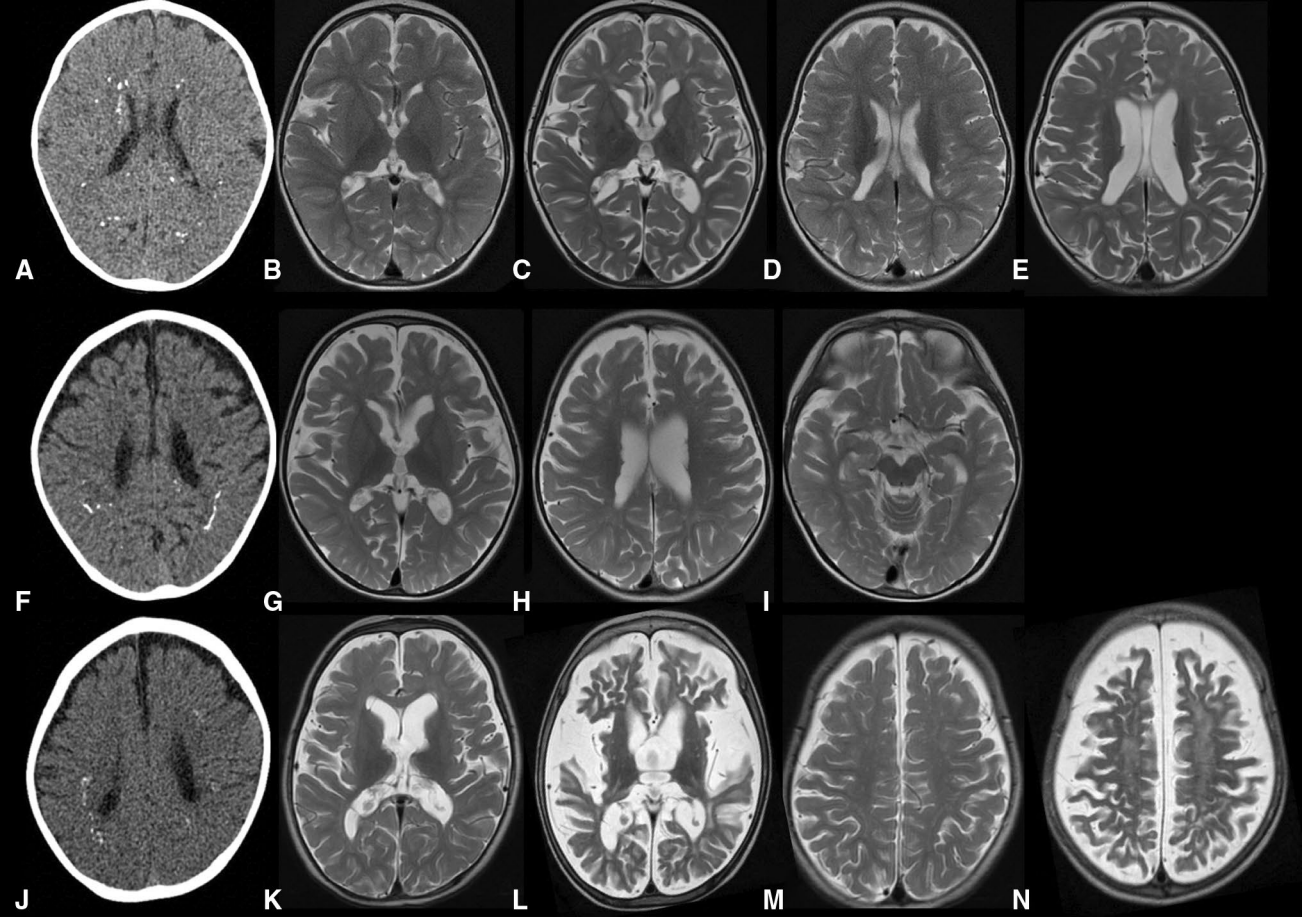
Neuroimaging of patients from F1442 and F2382. CT images of patient 1 (P1) aged 9 months (a), patient 2 (P2) aged 17 months (f), and patient 3 (P3) aged 14 months (j) demonstrate widespread spot and linear calcification in the deep and sub-cortical white matter. T2-weighted axial MR images of P1 aged 9 months (b, d) and 27 months (c, e), P2 aged 17 months (g, h, i), and P3 aged 14 months (k, m) and 27 months (l, n). Initial imaging shows mild cerebral volume loss with relatively good myelination. Follow-up shows rapidly progressive cortical and sub-cortical atrophy in P1 and P3. There is diffuse high signal in the white matter in P3 (l, n), but not P1
